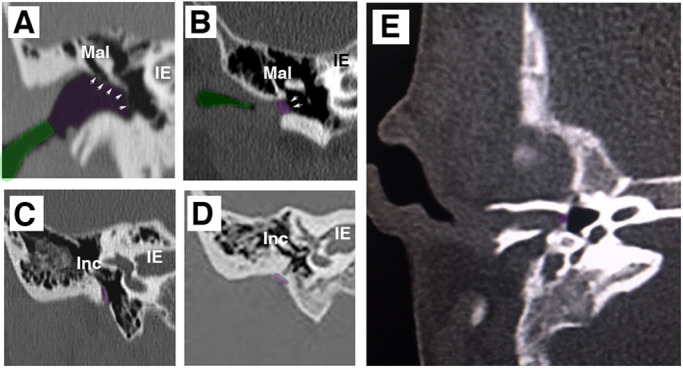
Scans of canal aplasia and atresia in patients. CT scans of patients. (A) Normal ear. (B-E) Patients with ear canal defects. (A) Coronal section through the ear showing a normal ear with a patent bony (purple) and cartilaginous (green) canal. The tympanic membrane is visible and outlined with arrows. (B) There is a stenotic lateral ear canal (green) and a medial meatal plug (purple). Arrows indicate the medial aspect of the metal plug at the tympanic annulus. (C,D) Patients have no evidence of a canal and the middle ear is closed off by a bony atretic plate (purple), which can be thin (C) or thick (D). (E) Patient with a closed ear canal. The bone still forms around the canal, suggesting that epithelial tissue is present. The primary canal is open near the pinna. IE, inner ear; Inc, incus; Mal, malleus.
